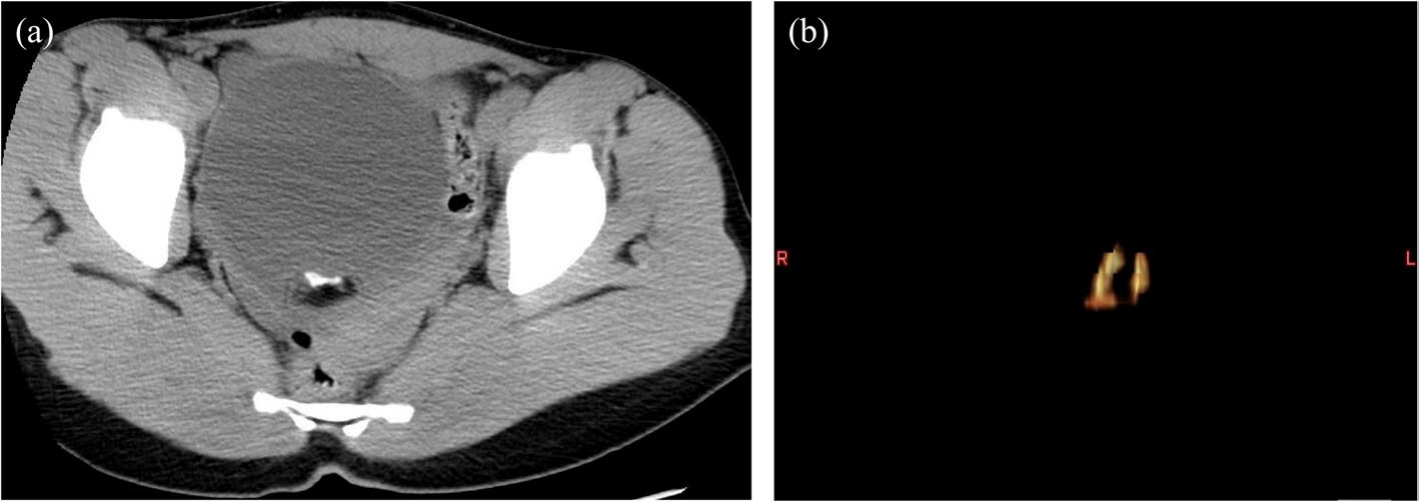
A 17-year-old female with mature cystic teratoma. a CT image shows the calcification in the tumor or cyst wall filled with sebaceous material. b A three-dimensional image shows a few small calcifications
